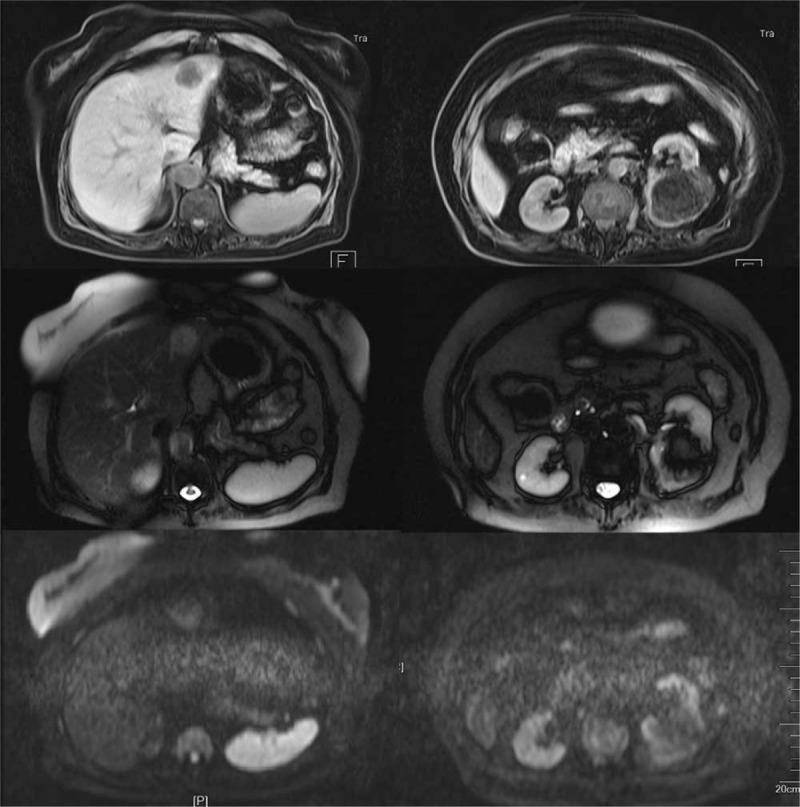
The MRI examination showed an approximately 8.4 × 5.8 cm well-demarcated mass with mixed signals in the lower pole of the left kidney, with low signal intensity on T1W imaging and slightly high signal intensity on T2W imaging. The masses of the left kidney considered to be an angiomyolipoma and the masses of liver need to exclude the possibility of a metastatic tumor. MRI = magnetic resonance imaging.
